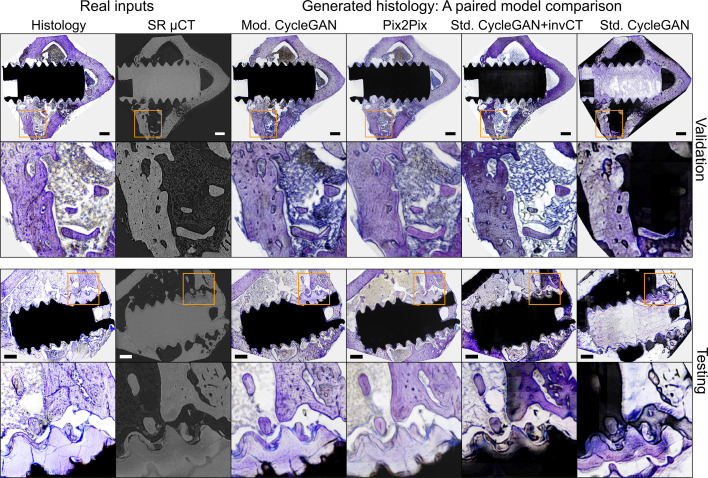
Inference results from each of the paired model variants tested, with WSI and 256 pixel ROI examples from validation and test data sets. ‘Mod. CycleGAN’ refers to the modified CycleGAN including the extra supervisory and greyscale loss terms, which is our chosen model. ‘Std. CycleGAN’ refers to the Standard CycleGAN model, which was trained from the same paired data sets, but without the extra loss terms. ‘Std. CycleGAN + invCT’ refers to a variant of the same model but trained and tested with an inverted CT input, in an attempt to combat the issue of intensity mismatch. The scalebar in each WSI represents 500 µm.
